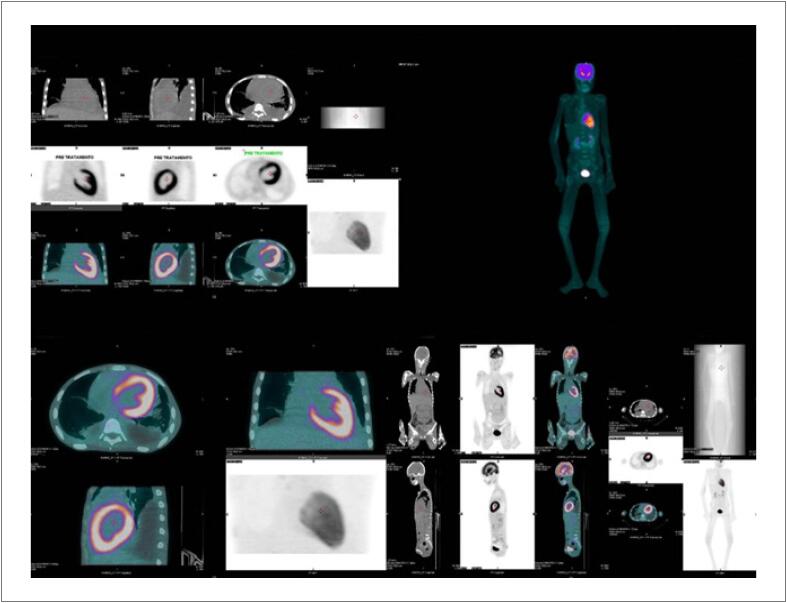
^18^F-FDG PET/CT na miocardite lúpica pré-tratamento: Observa-se intensa captação difusa de 18F-FDG no ventrículo esquerdo inferindo quadro de miocardite
